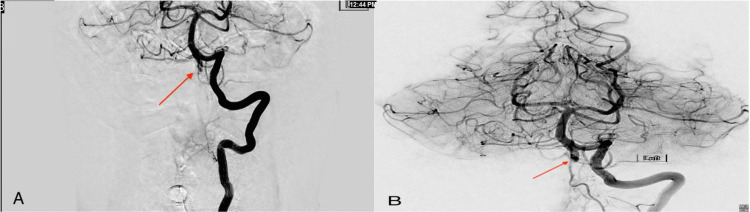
Computed tomography angiography. Patent vertebrobasilar junction and basilar apex with no evidence of large-vessel occlusion. There was a spontaneously recanalized distal right posterior cerebral artery occlusion and right cervical vertebral artery dissection.
